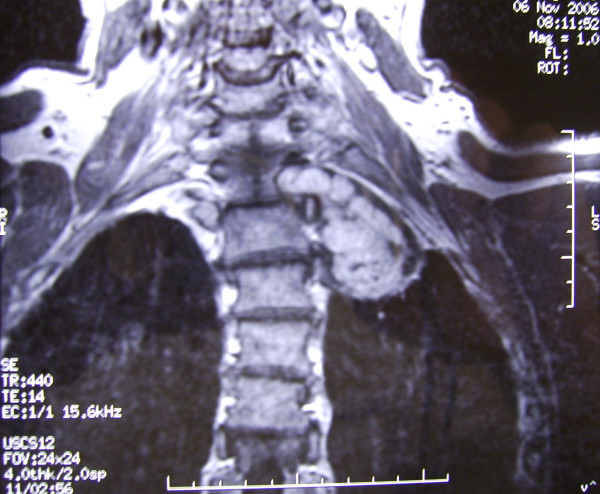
Coronal section of T1 weighted MRI demonstrating the left pulmonary apex tumor with extension into T1-2 intervertebral foramen.
